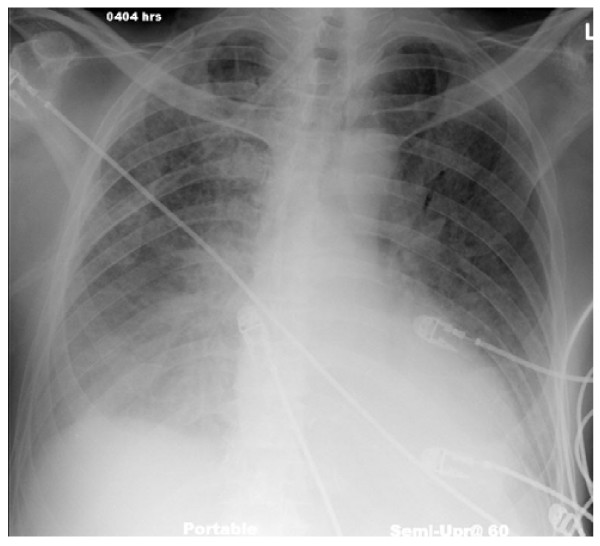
Portable AP chest radiograph of a 67 year old patient with pulmonary edema, small bilateral pleural effusions, and cardiomegaly five days post myocardial infarction.
